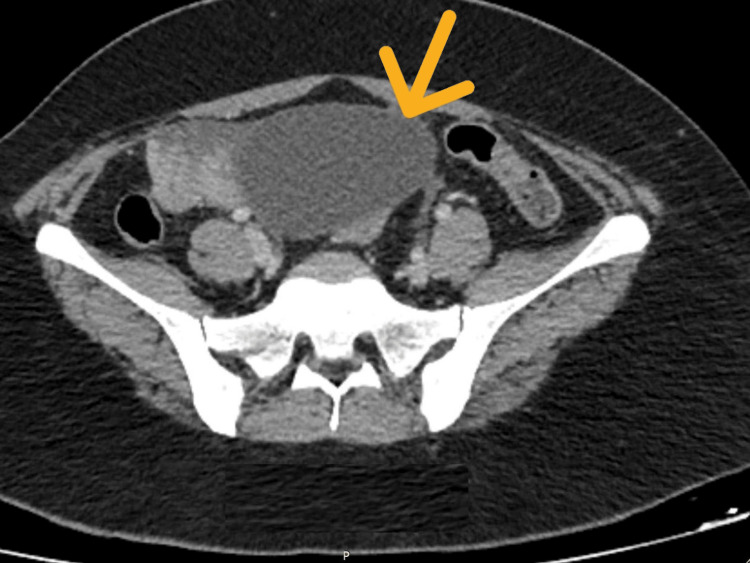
Craniocaudal cross-section of the distal ileal lesion (yellow arrow) on preoperative CECT. Arrow is pointed at the SCA of the ileum. The tumor appears hypodense relative to surrounding soft tissue. CECT: contrast-enhanced computed tomography
